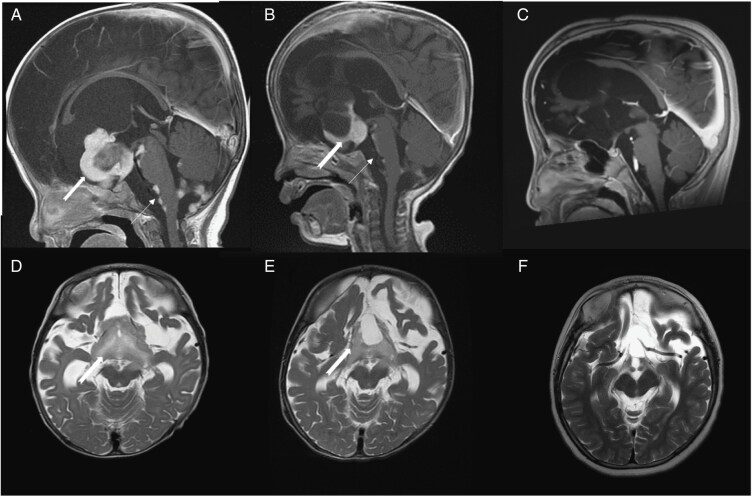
Image of a case that achieved a complete response after receiving carboplatin and vinblastine treatment. (A) T1-weighted postcontrast MR image. Sagittal view of the case prior to chemotherapy in June 2014. The Sella region OPHG is indicated by the thick arrow, and the area of multiple leptomeningeal dissemination is indicated by the thin arrow. (B) A sagittal image was taken 6 months later (after 6 cycles) following chemotherapy. The OPHG (thick arrow) shows a trend of reduction, and the leptomeningeal dissemination (thin arrow) also demonstrates a similar trend of decrease. (C) The most recent sagittal image was taken in September 2023. It shows no signs of recurrence since achieving a complete response. (D, E, F) T2-Flair MR image. Axial view from before treatment (D). six months later (E) and the most recent follow-up in September 2023 (F), which shows no recurrence.
